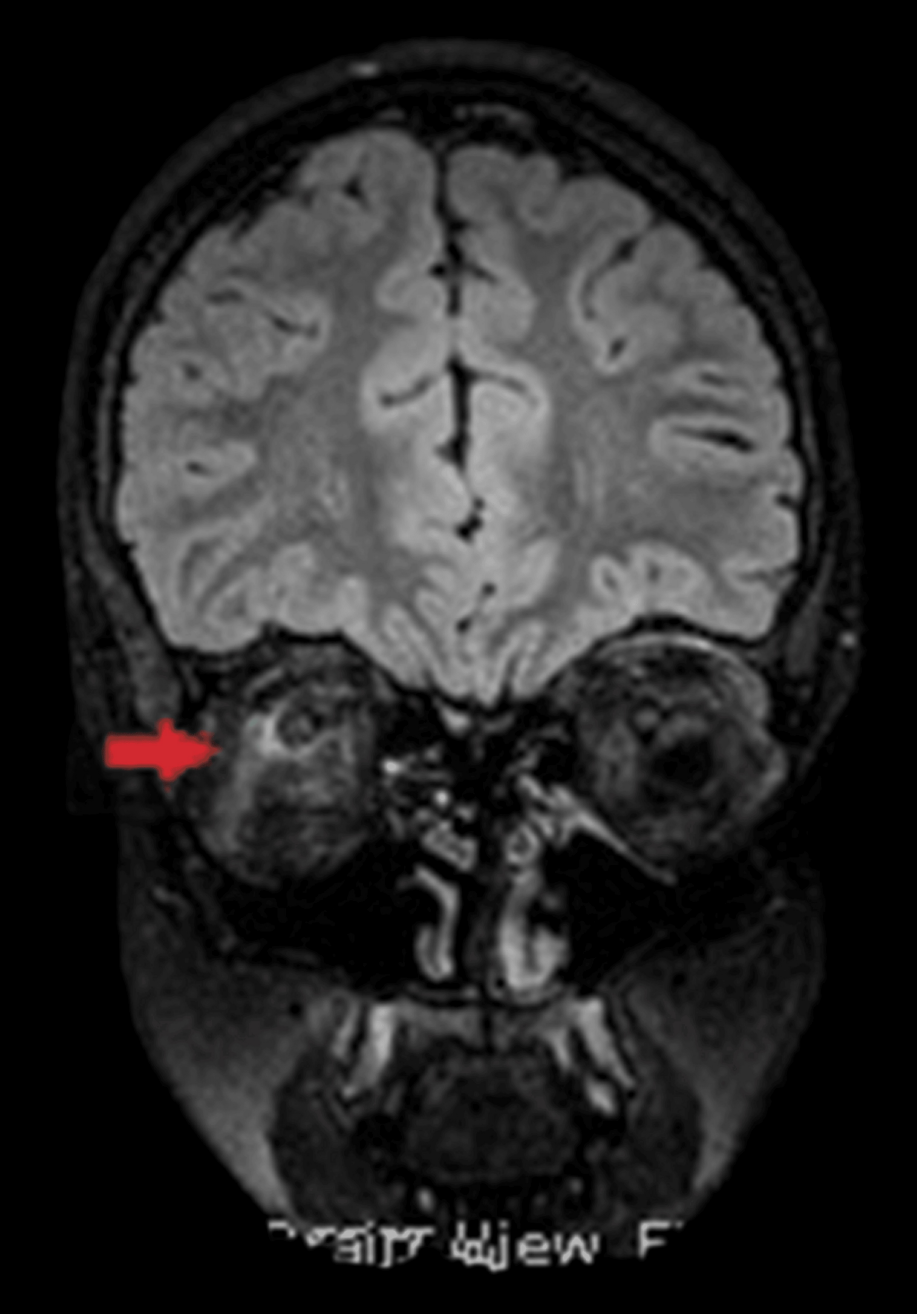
Coronal magnetic resonance imaging (MRI) of the orbits showing circumferential enhancement of the optic nerve sheath (Doughnut sign, indicated by red arrow).
